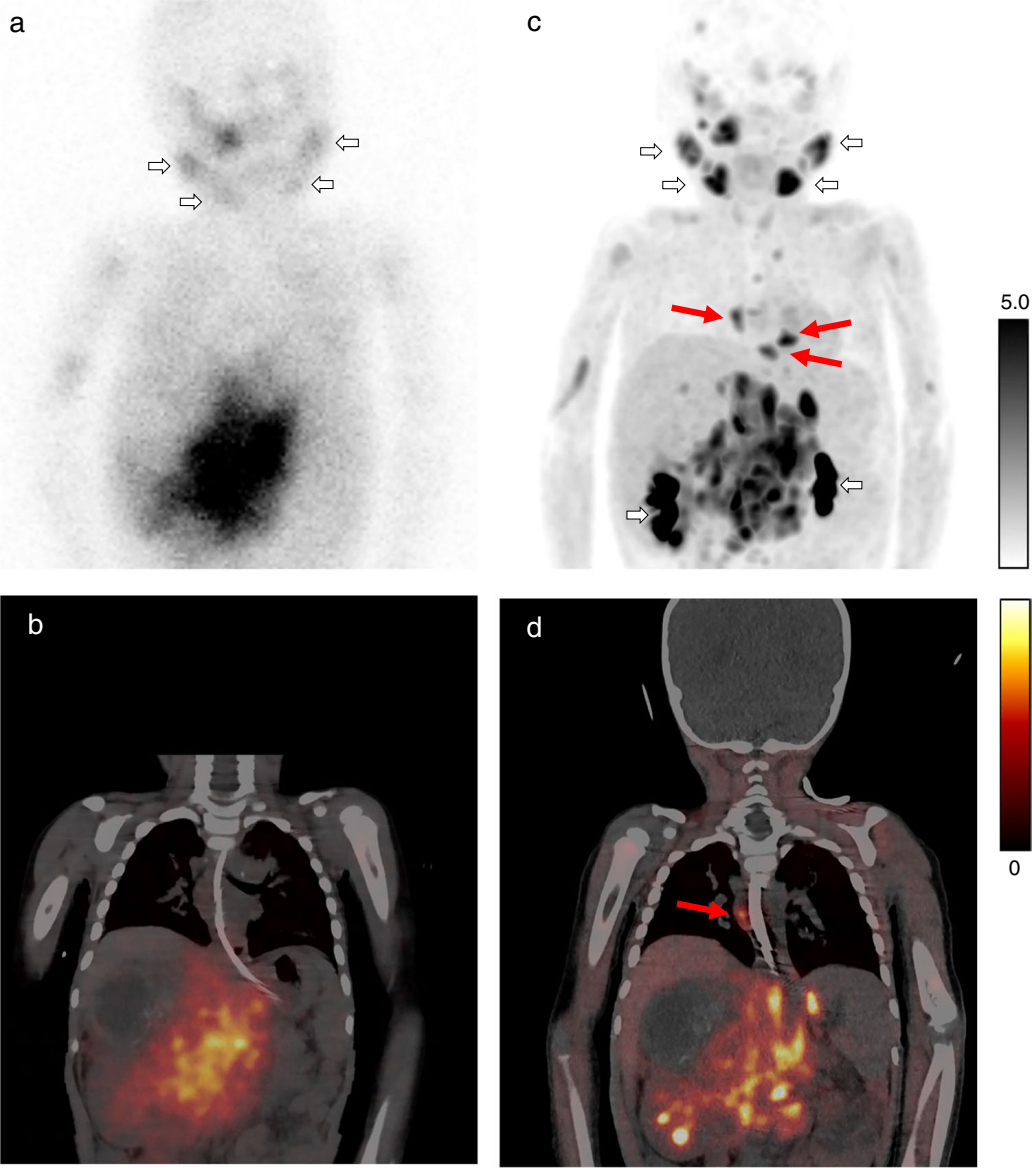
Planar [^123^I]mIBG scintigraphy (a) and fused single photon emission computed tomography (SPECT)-CT (b) and corresponding [^18^F]mFBG positron emission tomography (PET) maximum intensity projection (MIP) (c) and fused PET-CT (d) in patient 11 (scan pair 16). The short arrows (a and c) indicate physiological uptake (salivary glands and pelvicalyceal system), whereas other areas of uptake indicate tumour lesions. The long arrows (c and d) indicate additional mediastinal lymph node metastases detected on [^18^F]mFBG PET-CT but missed on [^123^I]mIBG scanning. Abbreviations: [^18^F]mFBG = *meta*-[^18^F]fluorobenzylguanidine, [^123^I]mIBG = *meta*-[^123^I]iodobenzylguanidine
